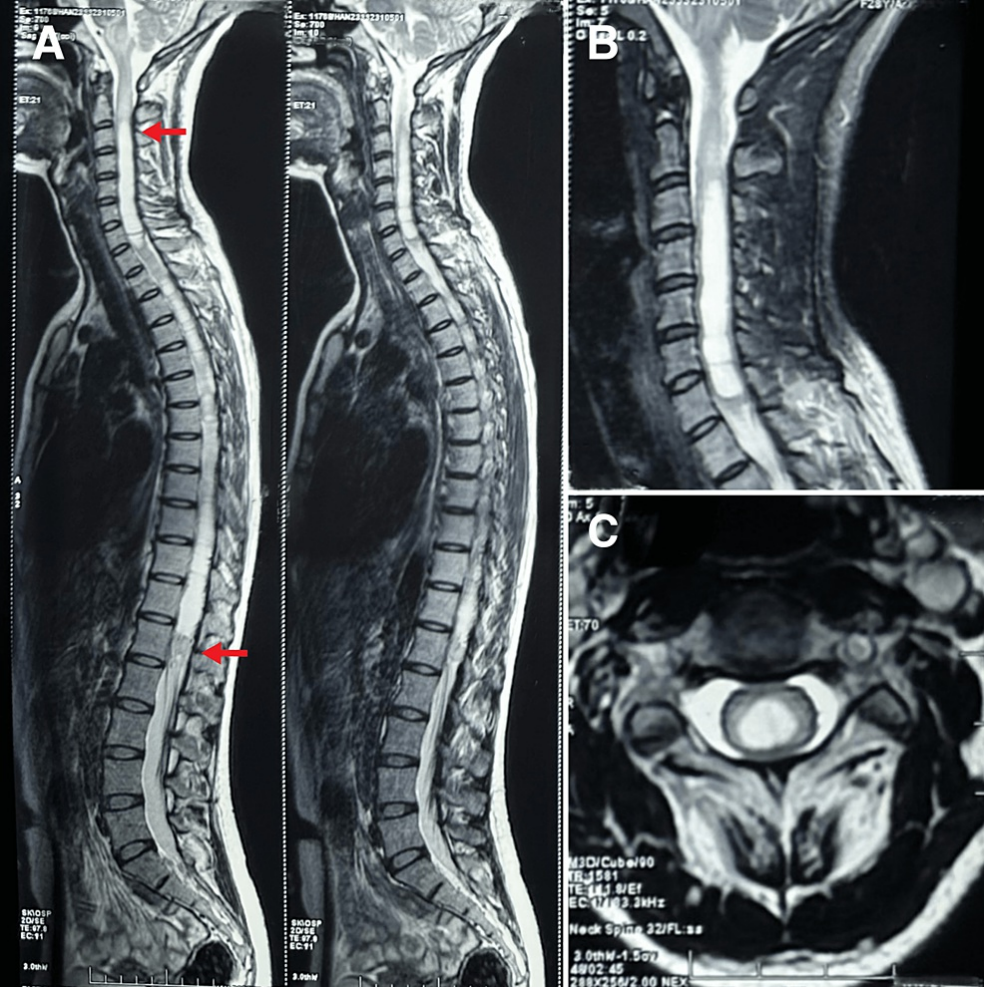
Magnetic resonance imaging (MRI). (A) A holocord syringomyelia from C2 to L1 (red arrows) and edema of the medulla and cervical cord above the syringomyelia. (B) Syringomyelia is observable at the sagittal view of the cervical spine. (C) A section showing hyperintense fluid in the spinal cord at the level of C3, which is consistent with a syrinx.
